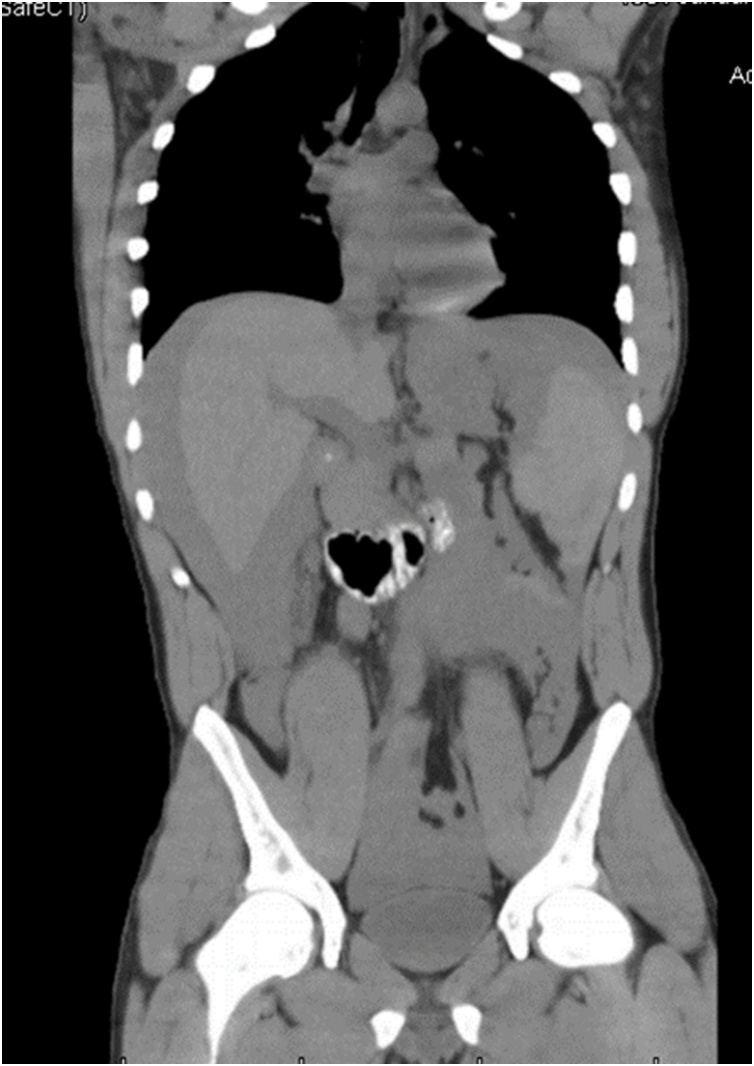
Coronal view of CT scan of chest, abdomen & pelvis.
